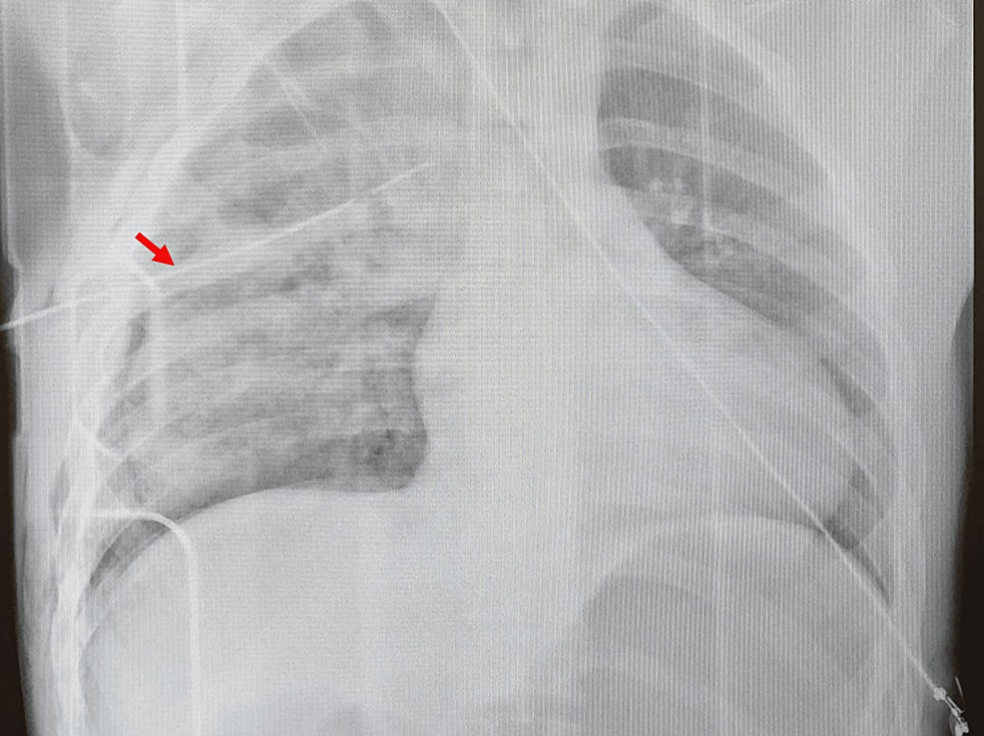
Chest X-ray after a right hemithorax decompression with a chest tube placed in the fourth intercostal space (red arrow).
